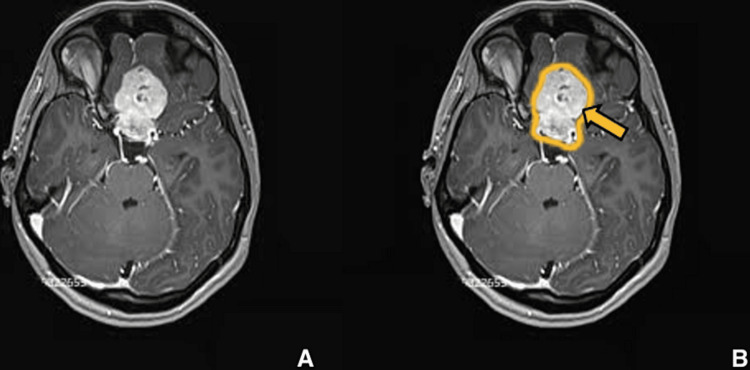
Preoperative imaging for patient 2. Contrast-enhanced T1-weighted axial image showing lesion involving sellar, suprasellar region measuring 3.6 x 4.7 x 8.5 cm. (A) Without ROI and (B) with ROI.
